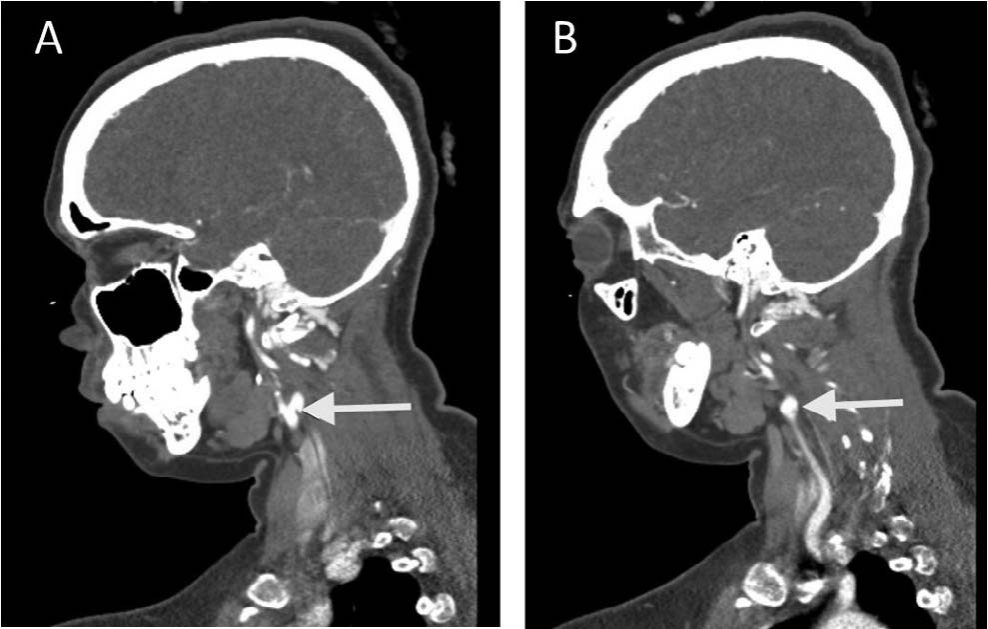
Computed tomography angiography images showing (A) the left carotid bifurcation and (B) the right carotid bifurcation.
